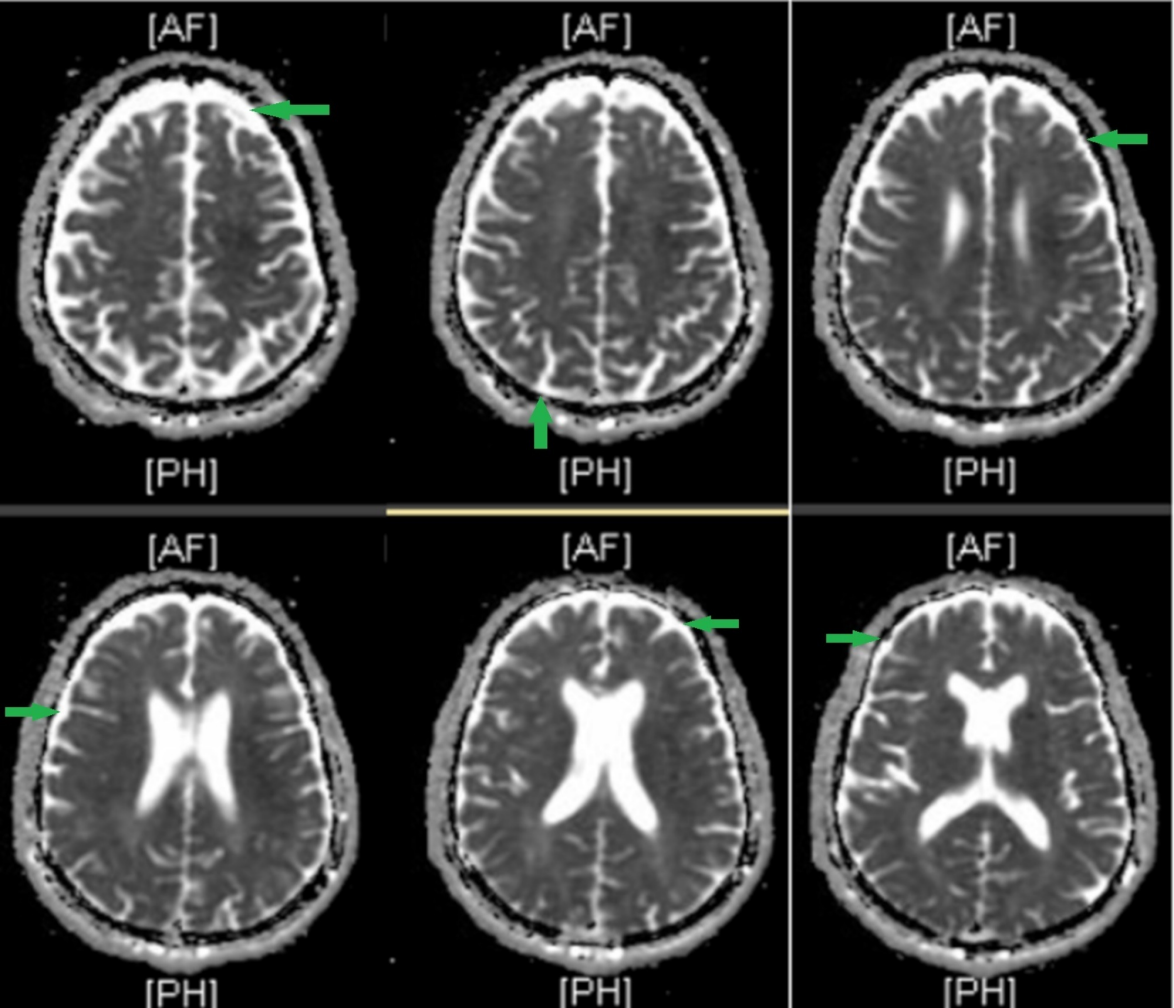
T2-weighted brain MRI of a 70-year-old male with neurosyphilis showing diffuse cortical atrophy. Green arrows indicate areas of cortical atrophy.
